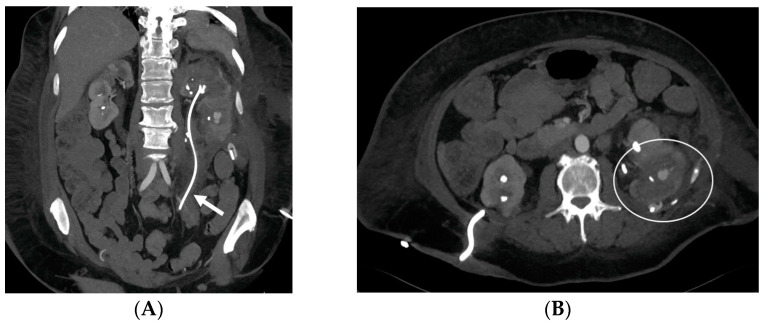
Vascular complication after double-J stent placement (arrow). CT arterial-phase images in the coronal (A) and axial (B) views demonstrate the presence of a pseudoaneurysm (circle) confined within a perirenal hematoma.
